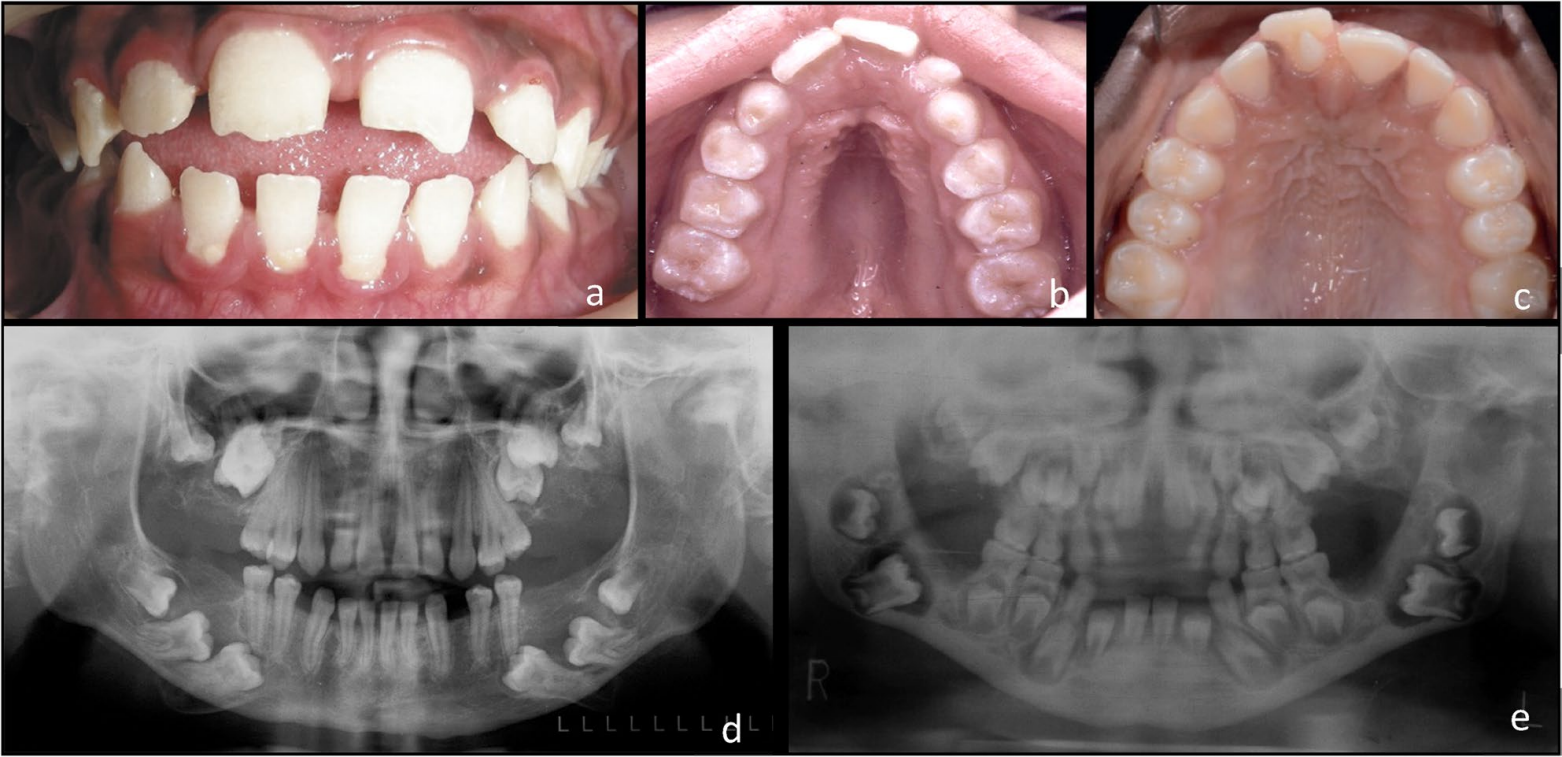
Representative clinical and panoramic radiographic findings in patients with mucopolysaccharidoses. (a) anterior open bite, (b) high arched palate, (c) talon cusp (d) thinning of mandibulaer cortex, impacted teeth, (e) short mandibular ramus, enlarged dental follicles. All images are anonymized and presented with informed consent
